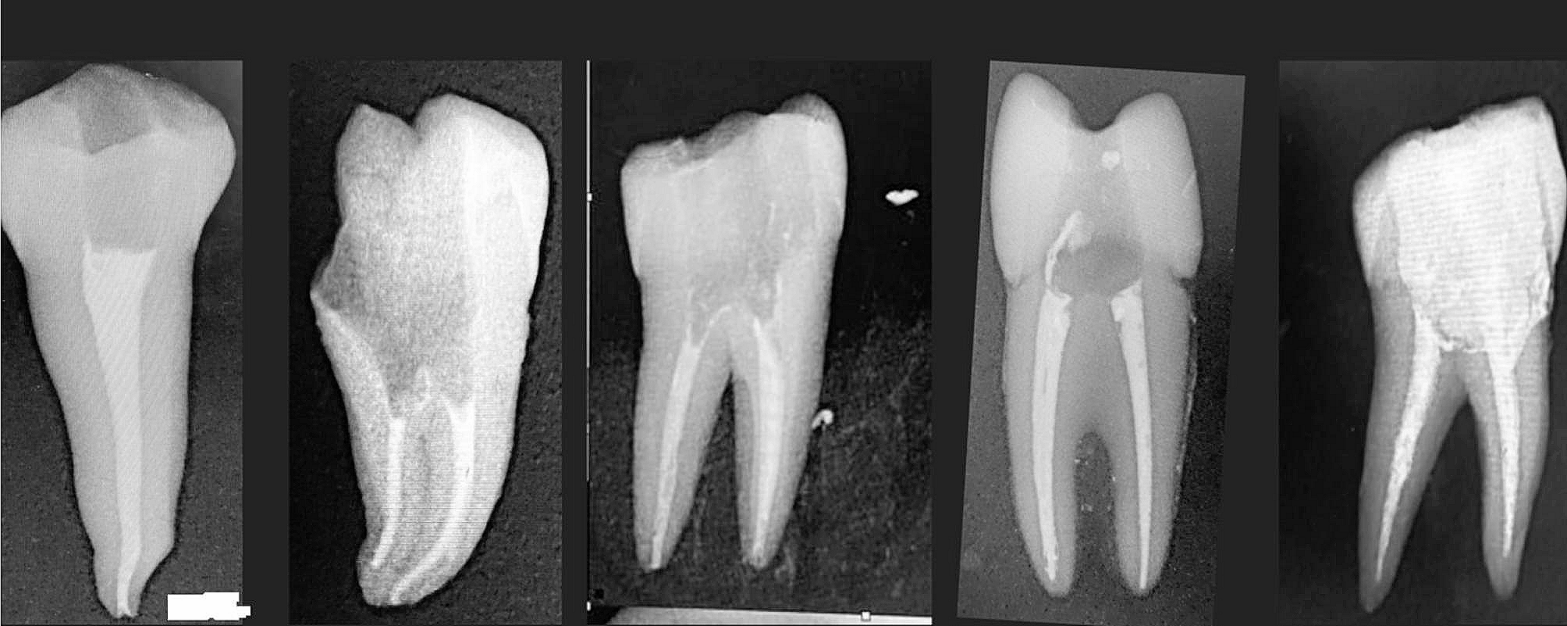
Some radiographic examples of the dental students work
